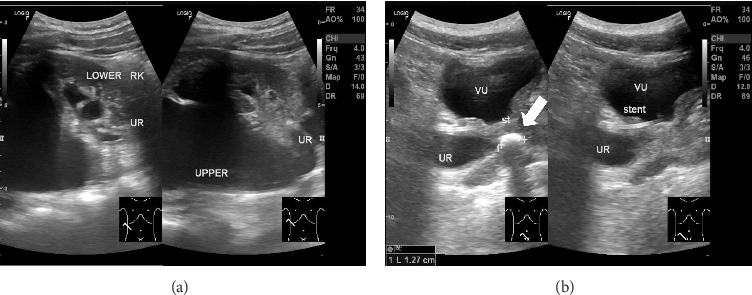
(a) Right kidney ultrasound showing: grade III hydronephrosis (upper moiety), grade I hydronephrosis (lower moiety), and grade I dilation of the lower moiety pelvicalyceal system (with ureteral stent in situ). (b) Right distal ureteric stone was observed originating from the upper moiety and ureteral stent was placed in the lower moiety.
